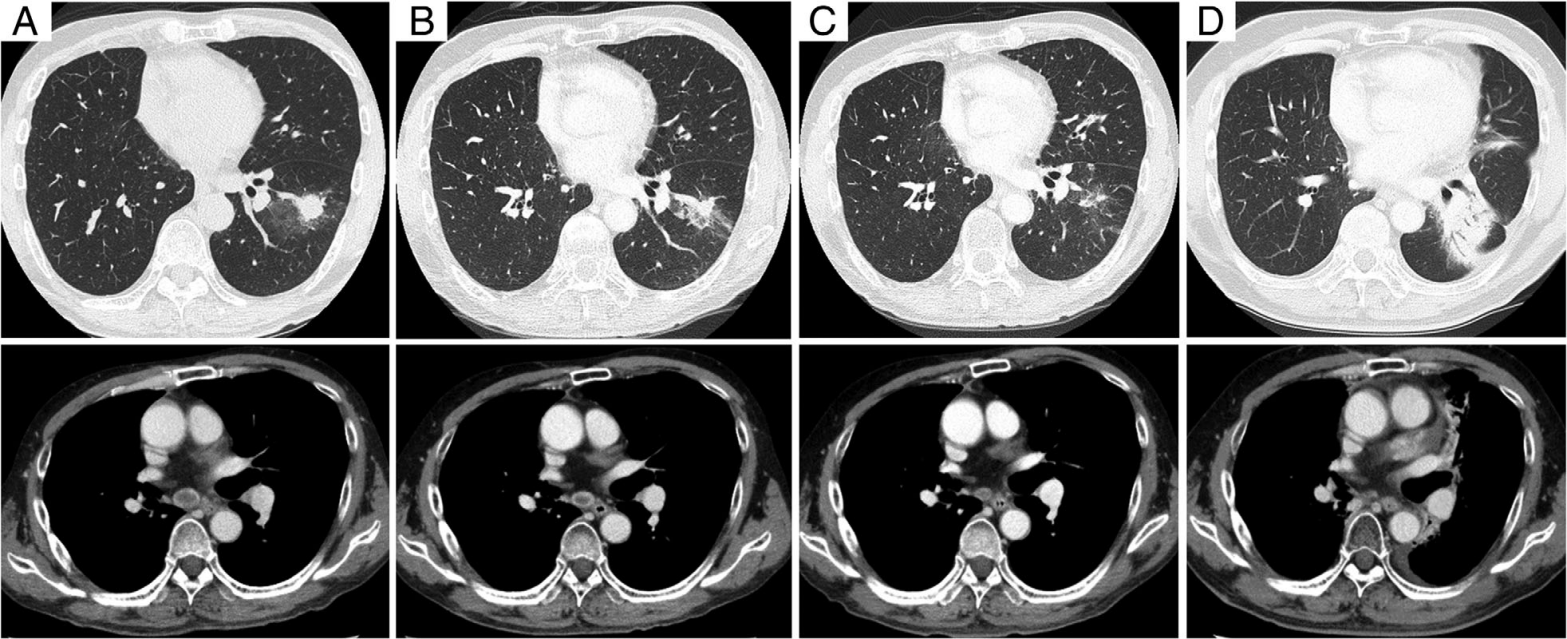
Imaging findings of chest computed tomography (CT) scans over time. (A) Before chemoradiotherapy; (B) at the end of chemoradiotherapy; (C) after the second course of durvalumab, when the size of the lung mass had decreased dramatically; and (D) after the 12th course of durvalumab, when grade I radiation pneumonitis appeared, while the mediastinal lymph nodes were still shrinking.
